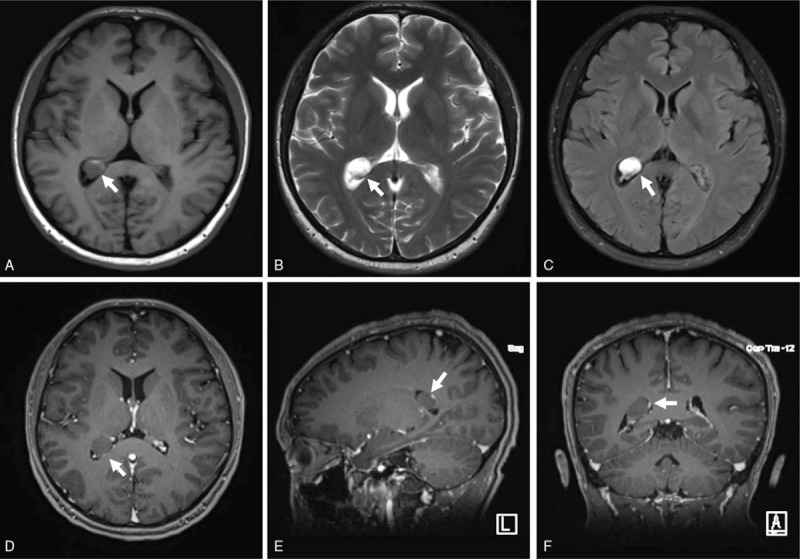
Preoperative magnetic resonance imaging showing a 1.3 × 1.2 × 1.0 cm oval mass filling the dilated posterior horn of the right lateral ventricle (white arrows). The lesion appeared hypointense on T1WI (A) including some hyperintense spots, and hyperintense on T2WI (B) as well as FLAIR images (C). Following contrast administration, no obvious enhancement was observed (D–F).
